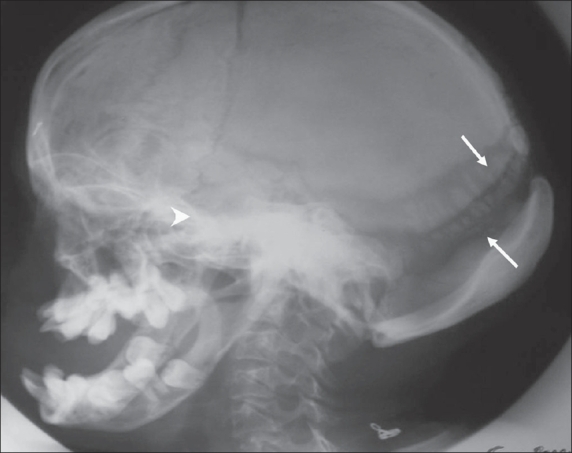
A lateral skull radiograph shows Wormian bones (arrows), widened sutures, an enlarged pituitary fossa (arrowhead), basilar invagination, and a hypoplastic frontal sinus
